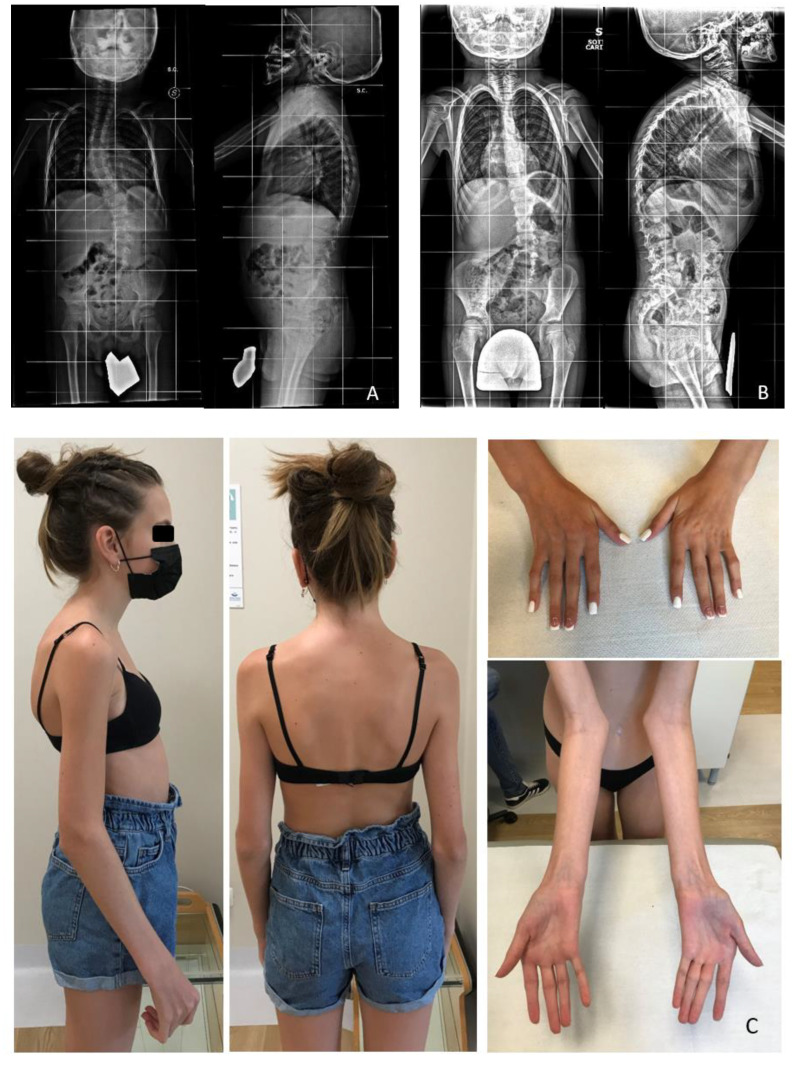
(A,B) include anteroposterior and lateral vertebral and pelvic X-Rays of patients 7 and 8 respectively at the age of 4 years old. It shows bilateral coxa valga, lumbar scoliosis, and thoracic kyphosis. (C) includes musculoskeletal findings of patient 14 with arachnodactyly, camptodactyly of fingers, simplified dermatoglyphic changes, ulnar deviation of the hands, restricted movements, pterygium like changes in elbows and neck, mild scoliosis, and moderate thoracic kyphosis.
